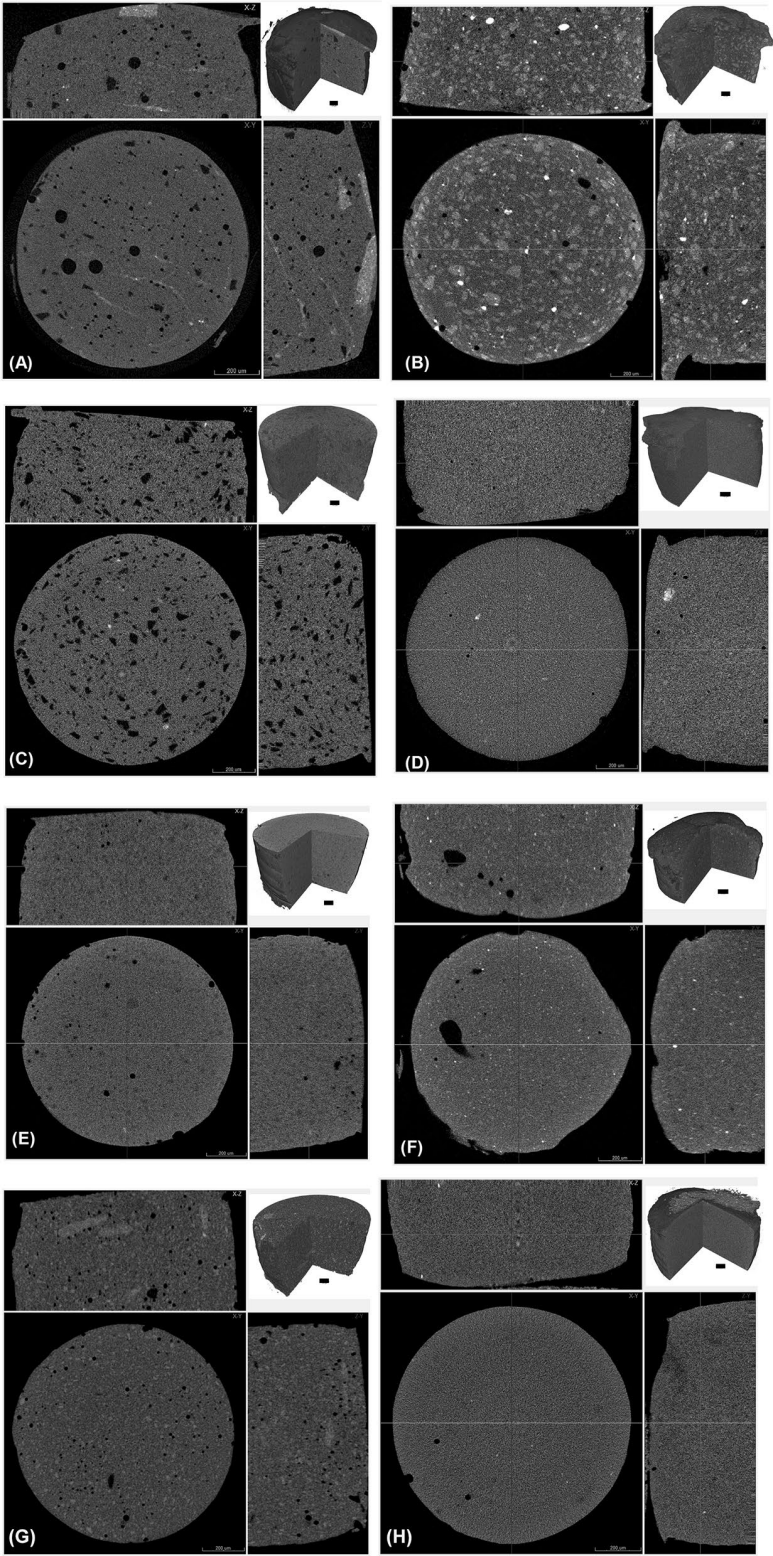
Nano-computed tomography: (A) Charisma, (B) Tetric EvoCeram, (C) Synergy D6, (D) Filtek Supreme XTE, (E) Ceram X, (F) IPS Empress, (G) Grandio nano, and (H) Venus. Images show a cross sectional slice scaled at 300 µm with an inset 3D image scaled at 100 µm.
